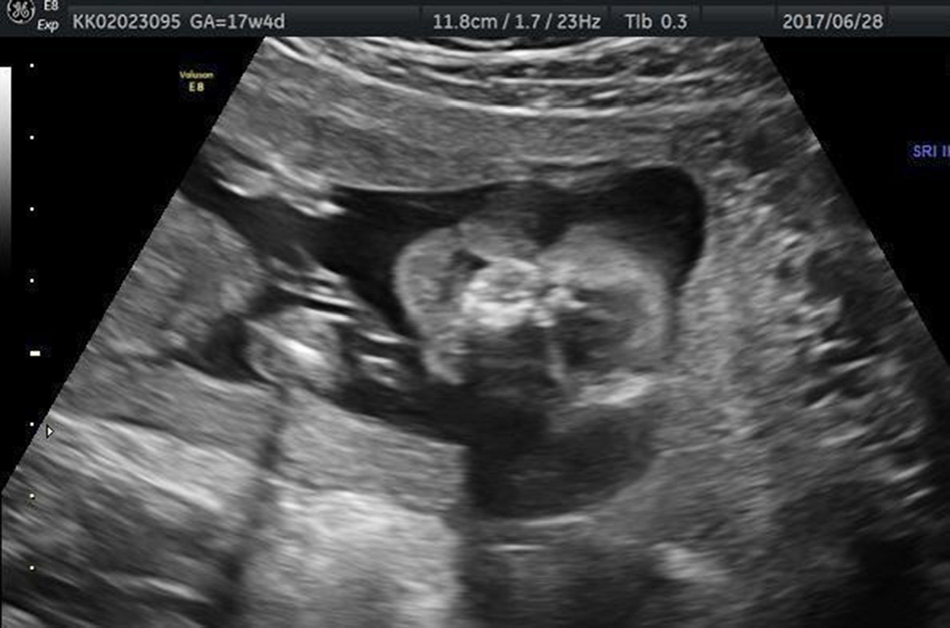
EEC syndrome. Two-dimensional ultrasound demonstrates the cleft lip.
